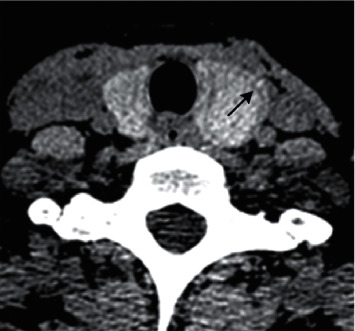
Thyroid left lobe nodule, in a female, 45 years old. Plain CT scan showing smooth low-density ring sign (↑) around the nodule, pathologically confirmed as nodular goiter.
